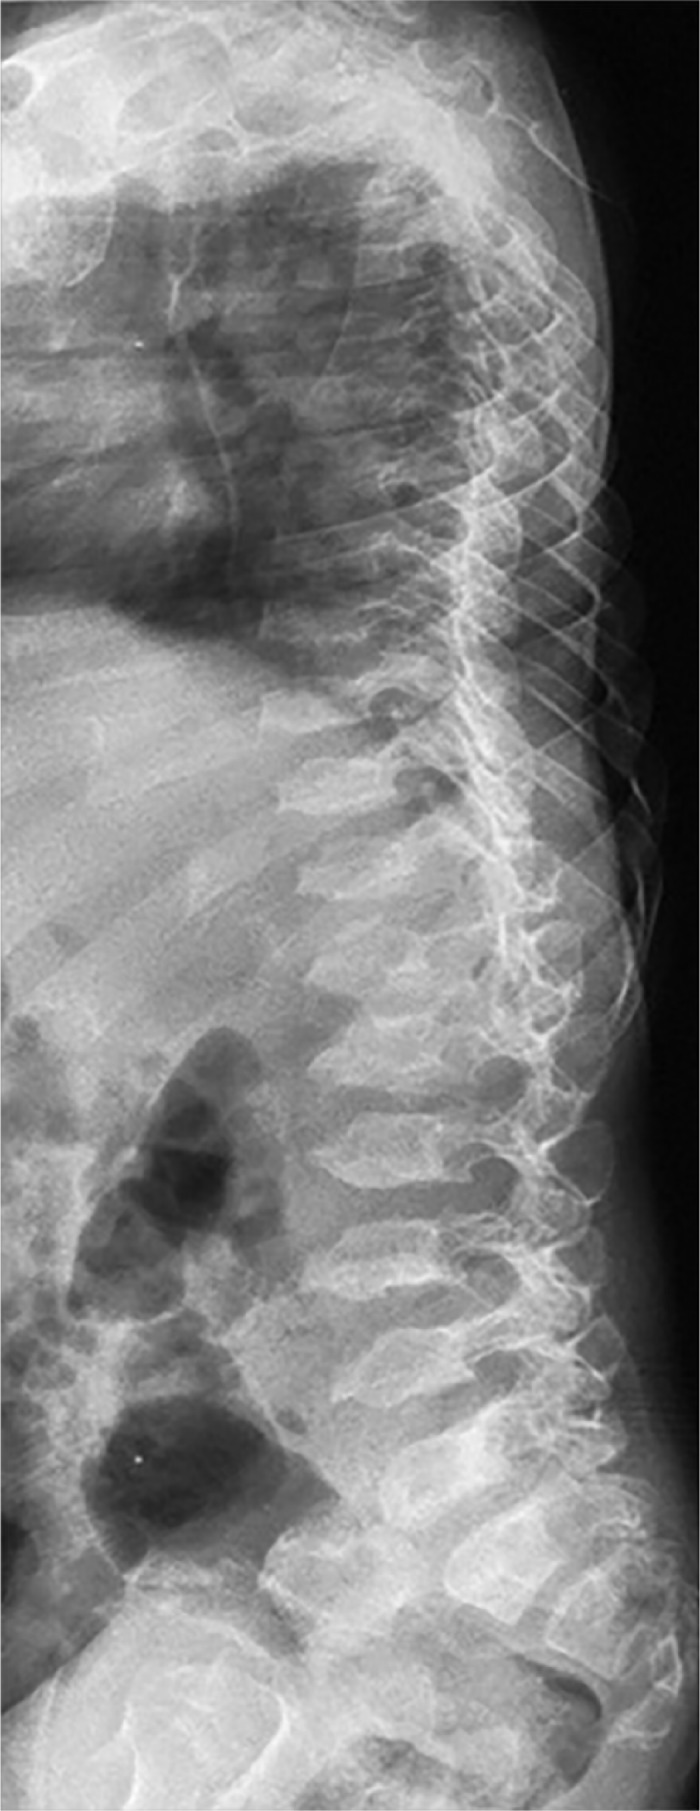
Lateral spine radiograph in a 3-year-old boy with MPS type IVA showed platyspondyly and oval-shaped vertebrae associated with dorsal wedging and deficient ossification of the anterior portions.
